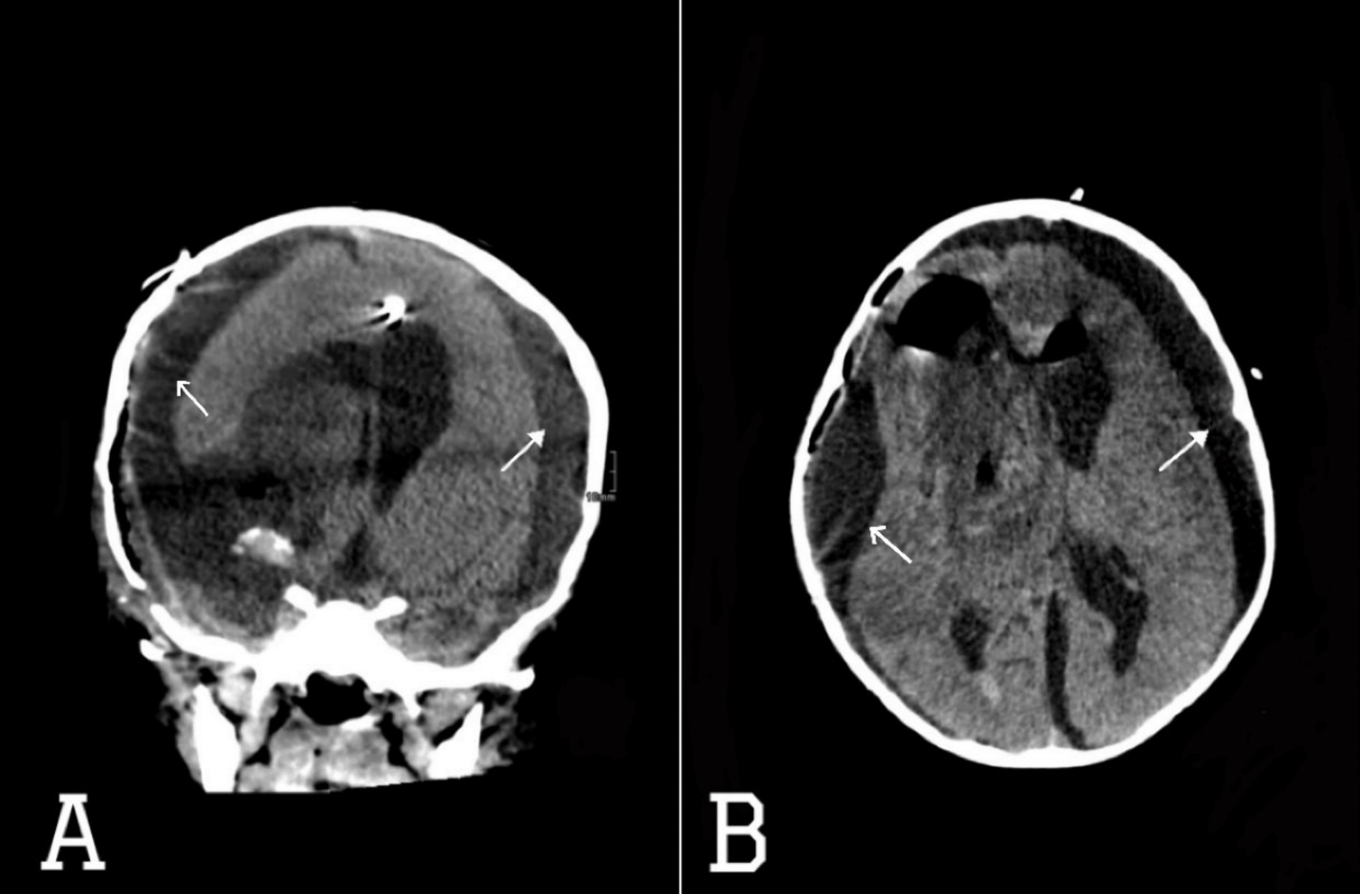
CT scan of the brain (A) axial and (B) coronal views showing postoperative changes of right frontoparietal craniotomy with newly developed left cerebral convexity subdural fluid collection of maximal thickness of 1.5 cm (white filled arrows) and interval increase in the right side subdural collection (white empty arrows), now measuring 1.8 cm compared to 1.3 cm previously. Interval decrease in size of the left lateral ventricle. Minor improvement of the left-sided midline shift and stable right uncal herniation.
